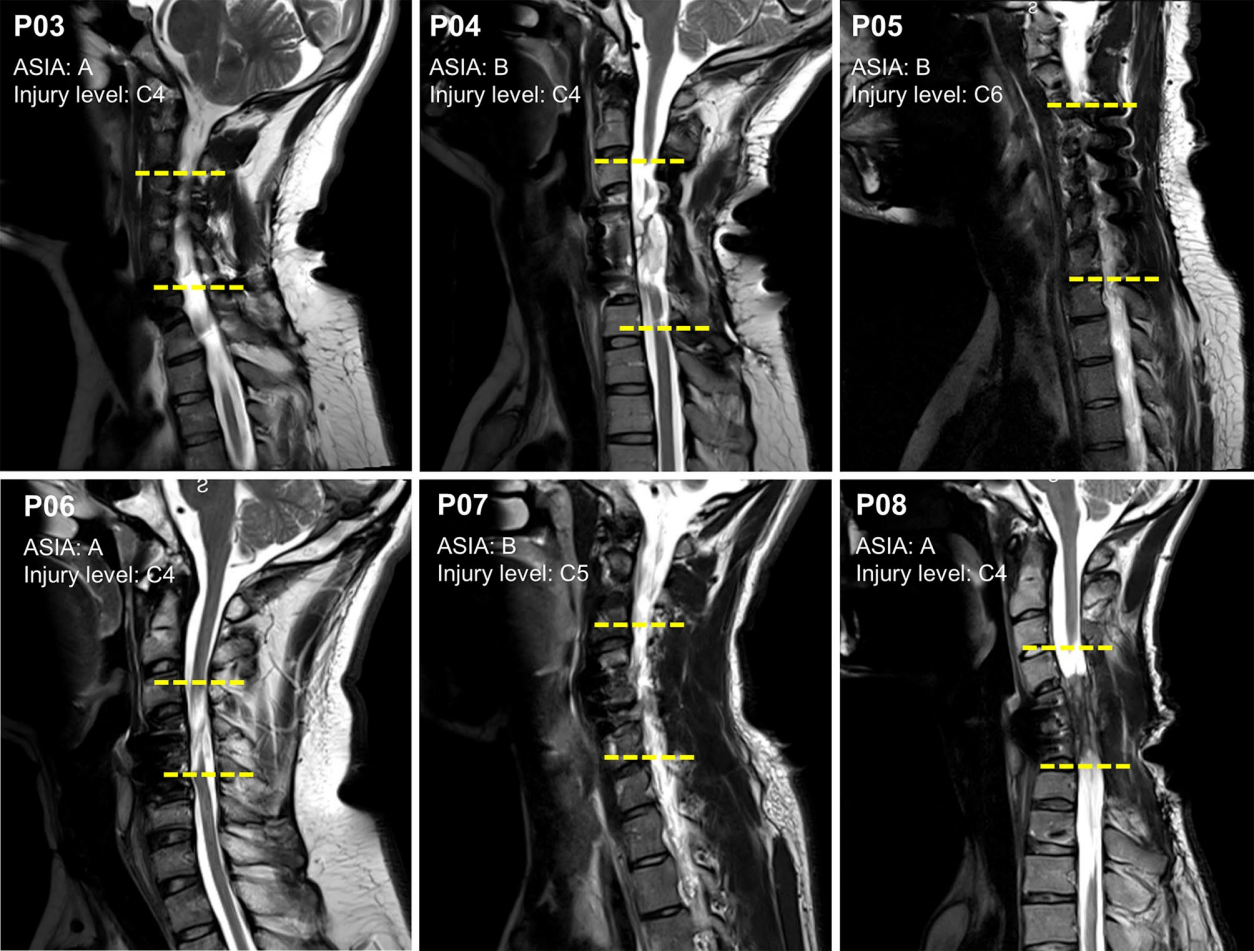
Structural magnetic resonance imaging (MRI) scans depicting sagittal sections within the cervical region. Sagittal slices from six participants with cervical spinal cord injury classified as AIS A and B. The yellow horizontal lines indicate the location of the cervical lesion between the upper and lower vertebrae.
